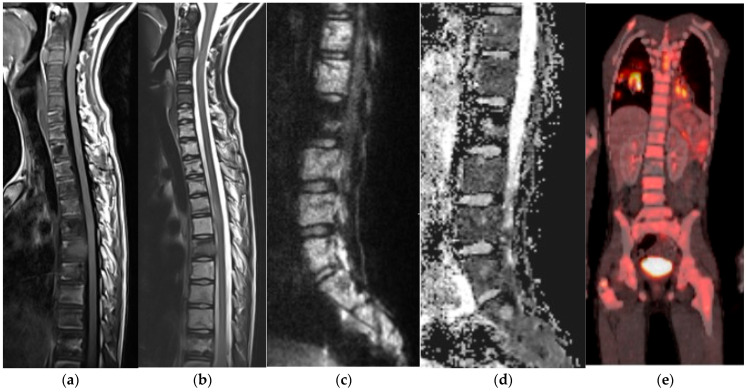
Lymphoma. (a) Sagittal T1 and (b) sagittal T2 MR, extensive marrow infiltrate with loss of normal fatty marrow signal on T1 and T2 sequences. (c) B-1000 and (d) ADC map MR sequences: restricted diffusion in the involved marrow helps diagnose lymphoma. (e) FDG PET/CT, tracer uptake in the bone marrow and right lung; PET helps with staging of lymphoma.
